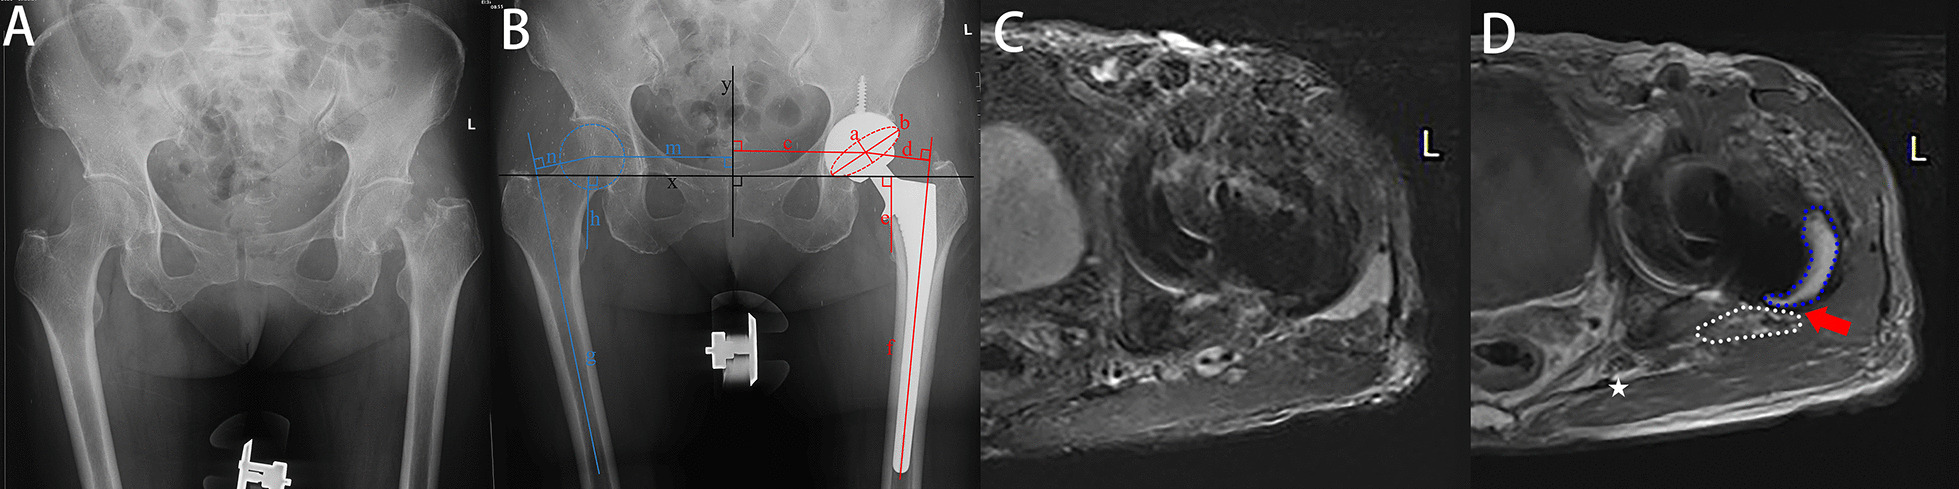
Female, 70 years old, femoral neck fracture (A). THA with mesh reconstruction was performed in this patient (B). Follow-up MRI (C, D) at six months after surgery showed that mesh was clung to the posterior femur without loosening sign (red arrows). The blue dashed line is the femur, the white dashed line is the mesh, the pentagram is the sciatic nerve. Detailed radiological measurements are shown in B acetabular anteversion angle = sin^−1^ (*a*/*b*); acetabular abduction angle = the angle between *b* and *x*; lower extremity length difference = *e* − *h*; offset difference = *c* + *d* − (*m* + *n*)
